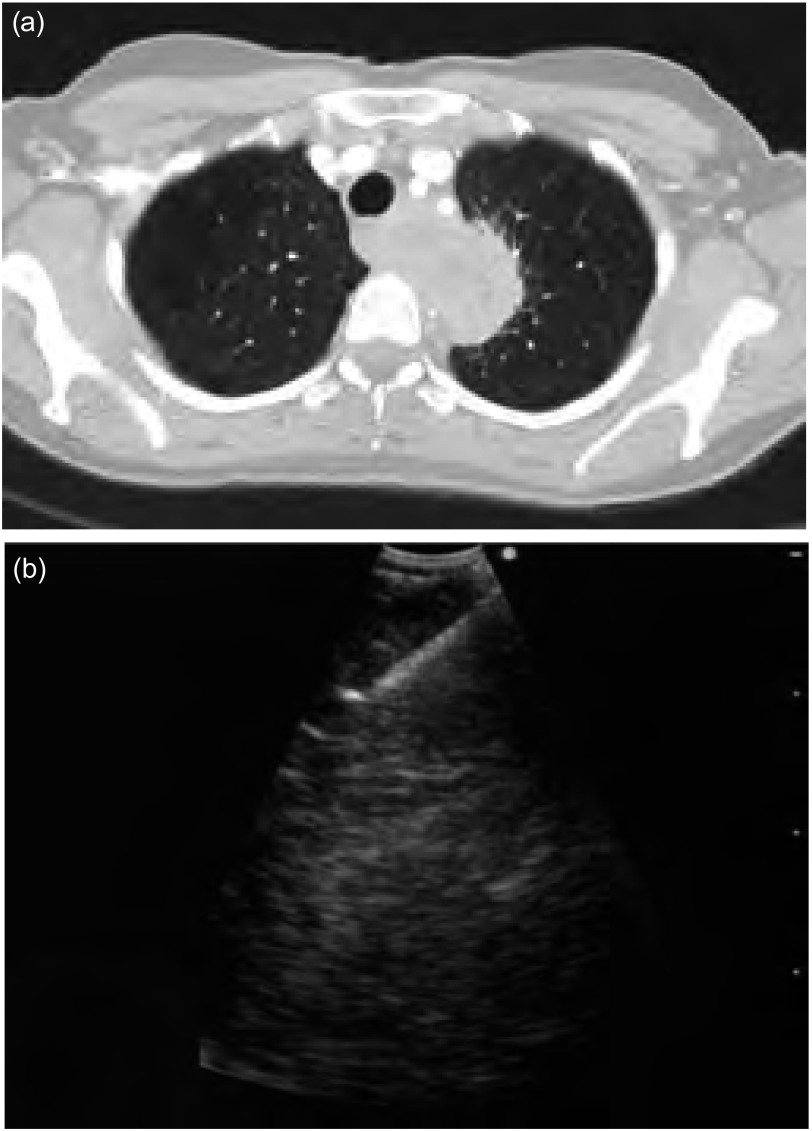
例3。（a）：胸部CT图像；（b）：超声图像，肿块占据图像大部，可见针吸活检通道。 Case 3. (a) Chest computerized tomography. (b) EBUS appearance showing the large mass occupying most of the ultrasound view, with EBUS-TBNA needle inserted for sampling.
